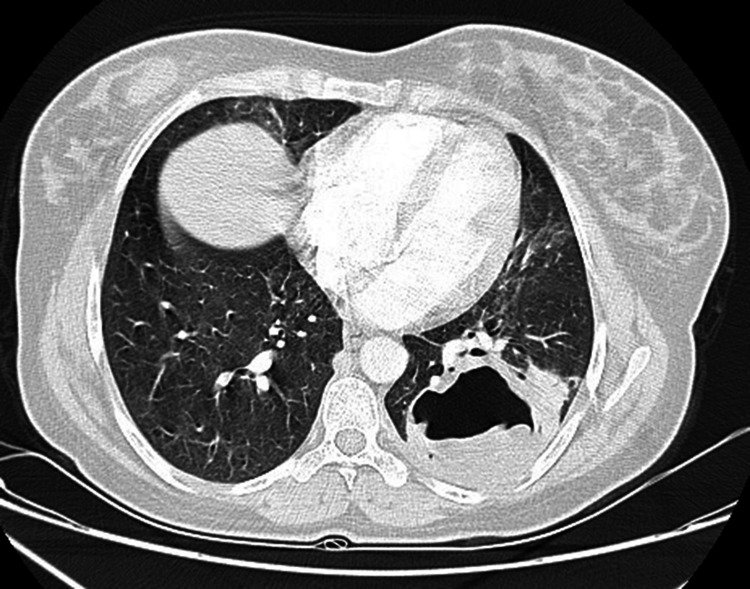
Axial contrast-enhanced chest CT image in mediastinal window showing a cavitary lesion of the left lower lobe communicating with the bronchial tree, strongly suggestive of a ruptured pulmonary hydatid cyst, with no sign of proximal pulmonary embolism.
